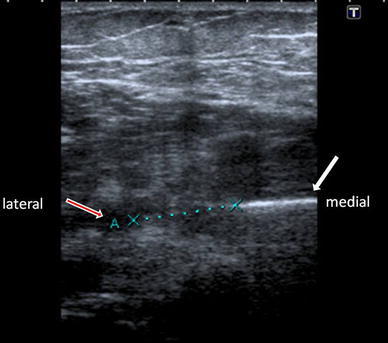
Ultrasound scan to monitor displaced meniscus with *medial arrow* showing position of dislodged meniscus and *lateral arrow* (*A*) indicating popliteal blood vessels
